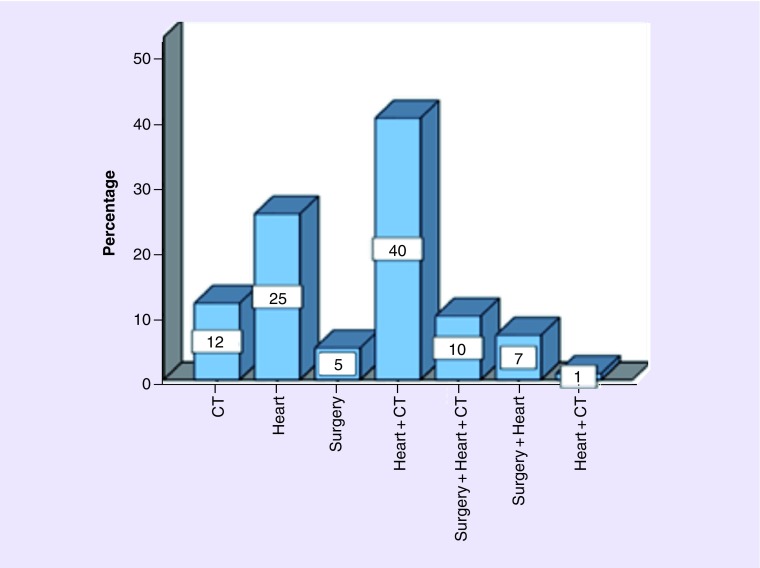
Treatment approach for brain metastases in the overall population. CT: Chemotherapy.
